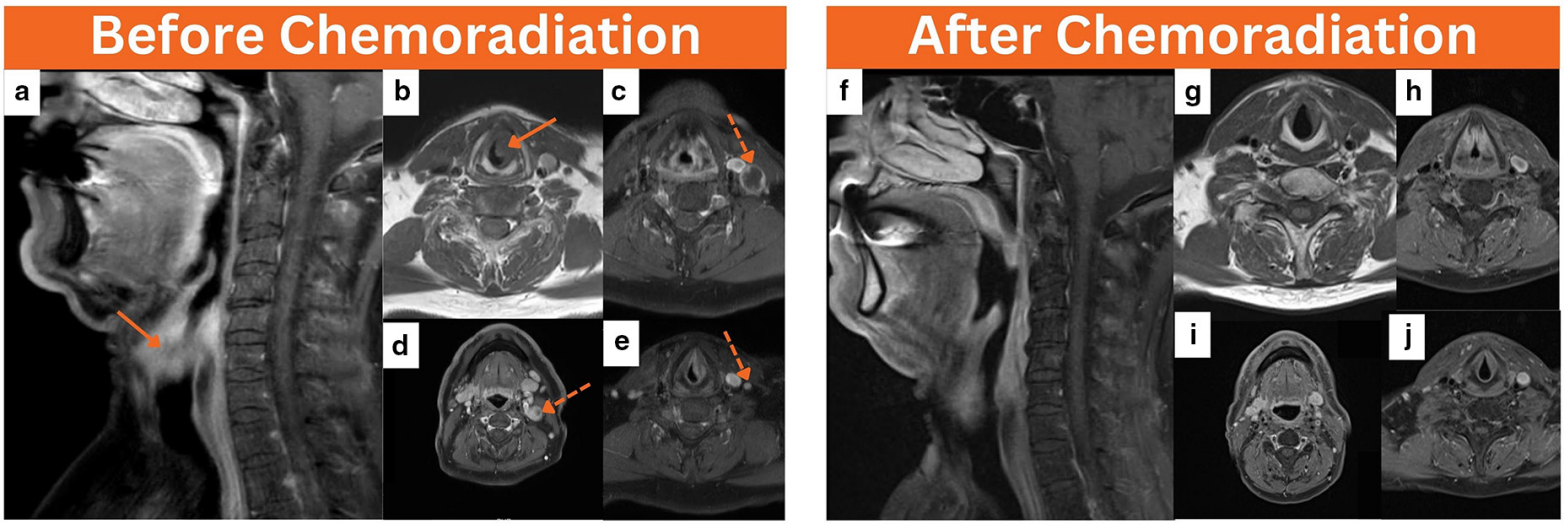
Baseline neck MRI was performed before chemoradiation, coronal T1 neck MRI (a) and axial T1 images (b) revealed a left transglottic laryngeal mass infiltrating the left paraglottic space and measuring about 2.5 cm in maximum dimension (arrows). In addition, axial T1 images of the neck (c–e) showed few concurrent ipsilateral enlarged level II–IV cervical lymph nodes, the majority of which demonstrate central necrosis (dotted arrows). At the end of therapy, coronal T1 neck MRI (f) and axial T1 neck images (g–j) concluded resolution of the previous locoregional disease.
